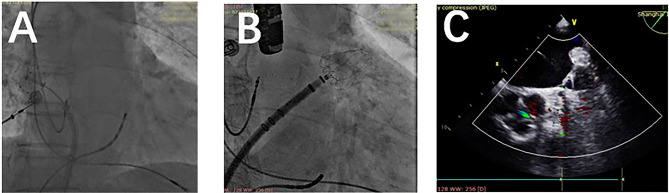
Fluoroscopy and transesophageal echocardiography (TEE) during the combined cryoballoon ablation (CBA) procedure. (A) During CBA, an inflated cryoballoon during the ablating of the left superior pulmonary vein. (B) Deploying a WATCHMAN device during left atrial appendage closure. (C) TEE confirmation of complete sealing stable positioning.
